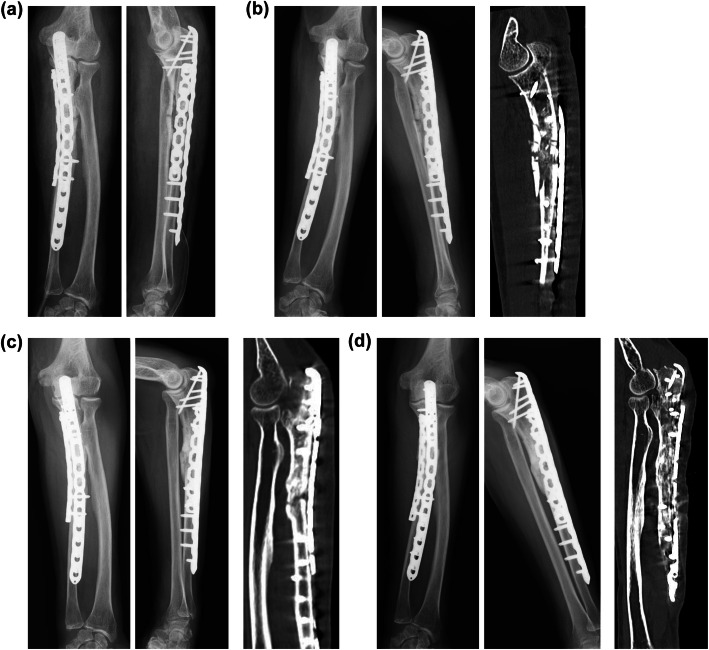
Postoperative radiography and computed tomography. a Post-operation (both panels, radiography). b 1 month after surgery (left two panels, radiography; right panel, computed tomography). c 6 months after surgery (left two panels, radiography; right panel, computed tomography). d 11 months after surgery (left two panels, radiography; right panel, computed tomography)
